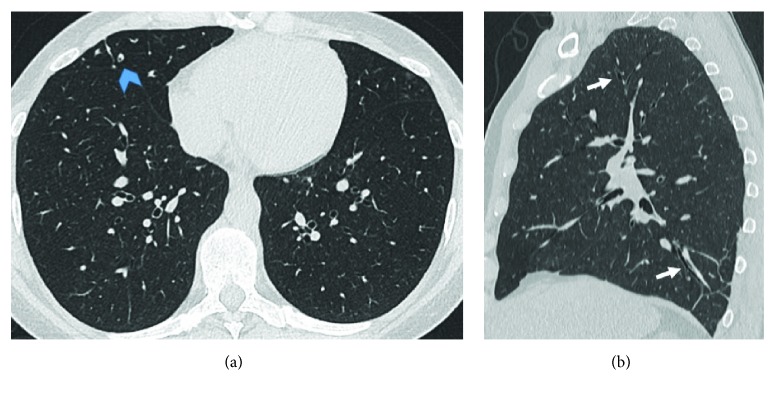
Axial HRCT scan (a) and sagittal reconstruction (b) show bilateral bronchiectasis (arrows) and some small mucoid impactions (blue arrowhead).
